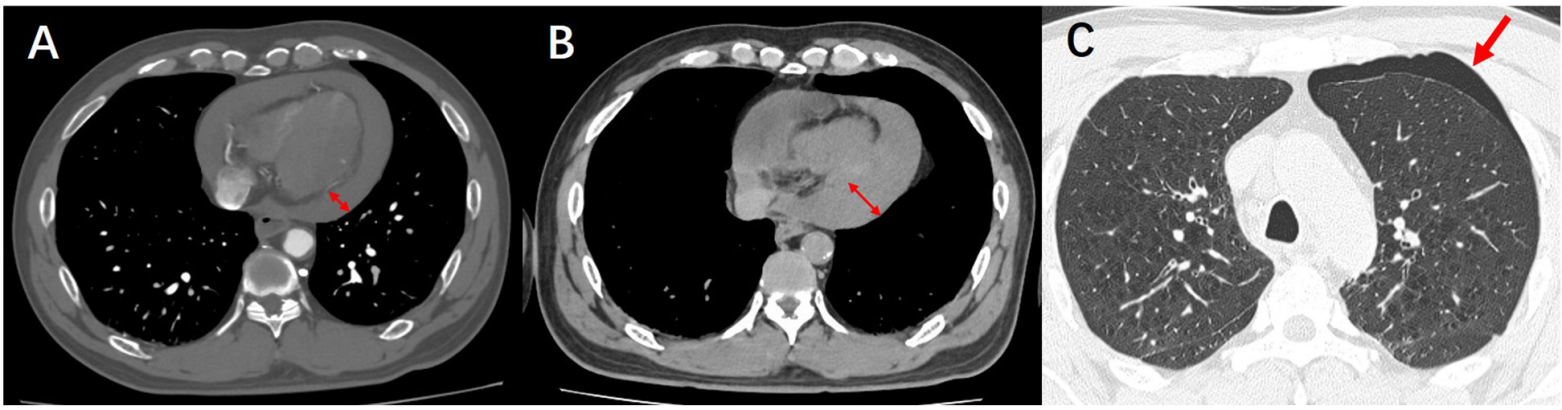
(A) CTA demonstrates pericardial effusion. (B) Follow-up lung CT reveals an increased volume of pericardial effusion. (C) Follow-up lung CT shows newly developed pneumothorax.
